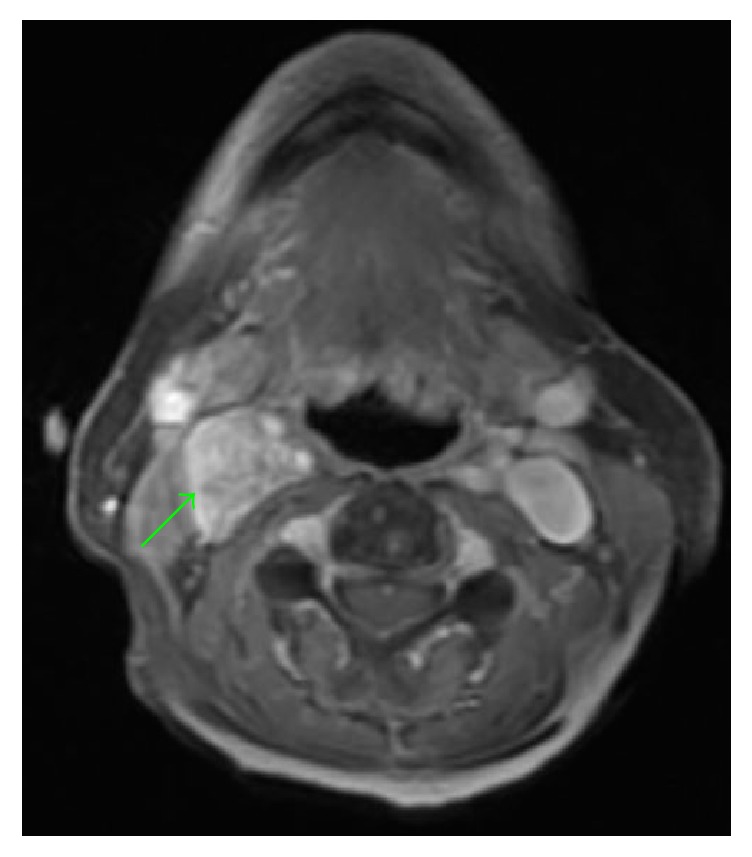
MRI, axial section, fast spoiled gradient echo with contrast. The green arrow refers to the neck mass.
